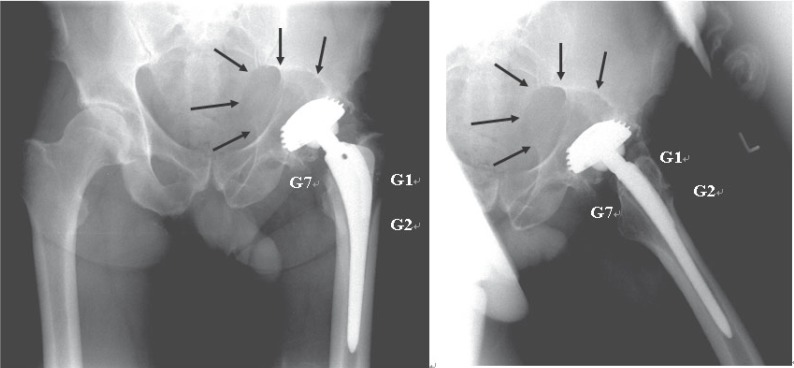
The X-rays of the pelvis in anterior-posterior (a) and axial planes (b) showed a thread cup with a large, expansive critical bony defect corresponding to a wear induced osteolysis. Moreover there are slight radiolucent lines at the proximal femur in Gruen-zones 1 (G1), 2 (G2) and 7 (G7).
